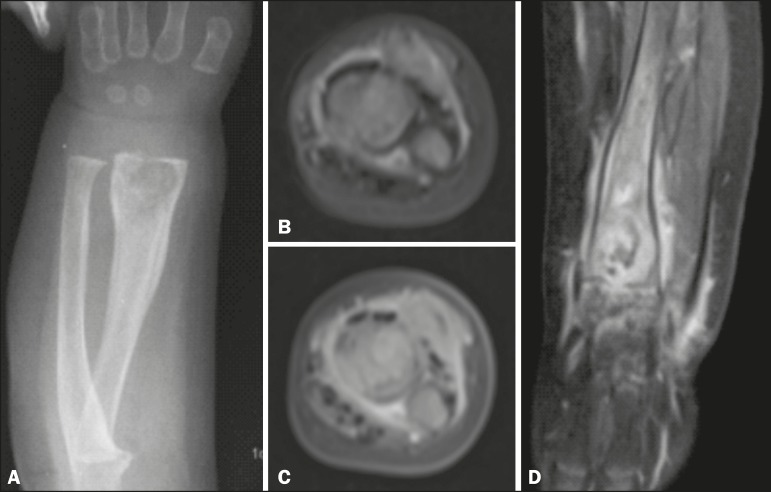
A: Anteroposterior X-ray of the forearm. Round osteolytic
